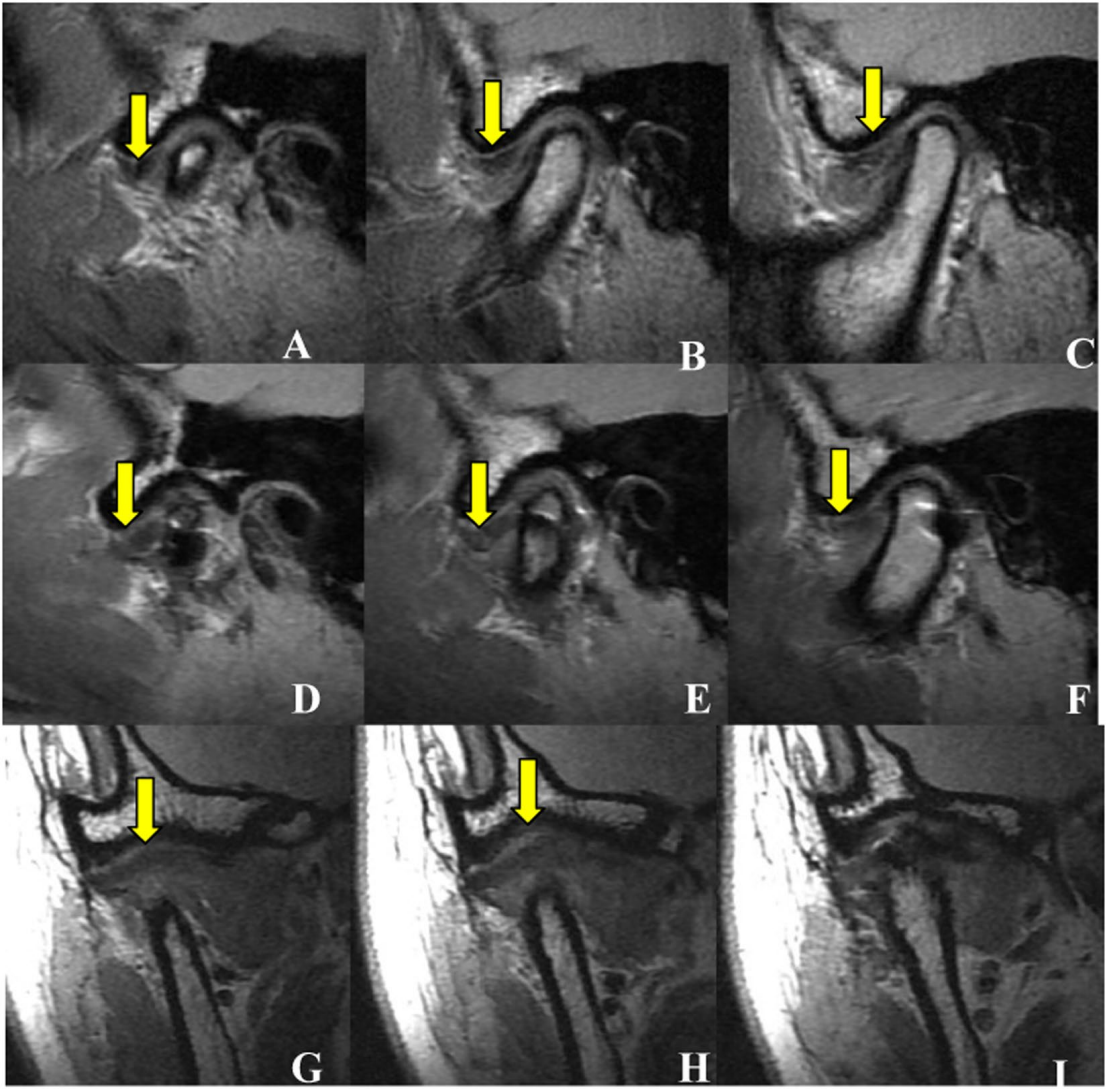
Disc position on MRI before and after operation – example of C classification. Internal (A), middle (B) and lateral (C) layers of the long axis of the condylar process shown before surgery, in sagittal plane. The images demonstrate that the disc is anteriorly displaced in the closed-mouth position (yellow arrow). Internal (D), middle (E) and lateral (F) layers of the long axis of the condylar process after operation, in sagittal plane. The images demonstrate that the displaced disc is not repositioned (yellow arrow). Anterior (G) and middle (H) layers of the long axis of the condylar process after surgery, in coronal plane. The images demonstrate that the displaced disc is not repositioned (yellow arrow), and in the posterior (I) layer the displaced disc is not found. As the disc is not re-set in any layers, the case was classified C.
